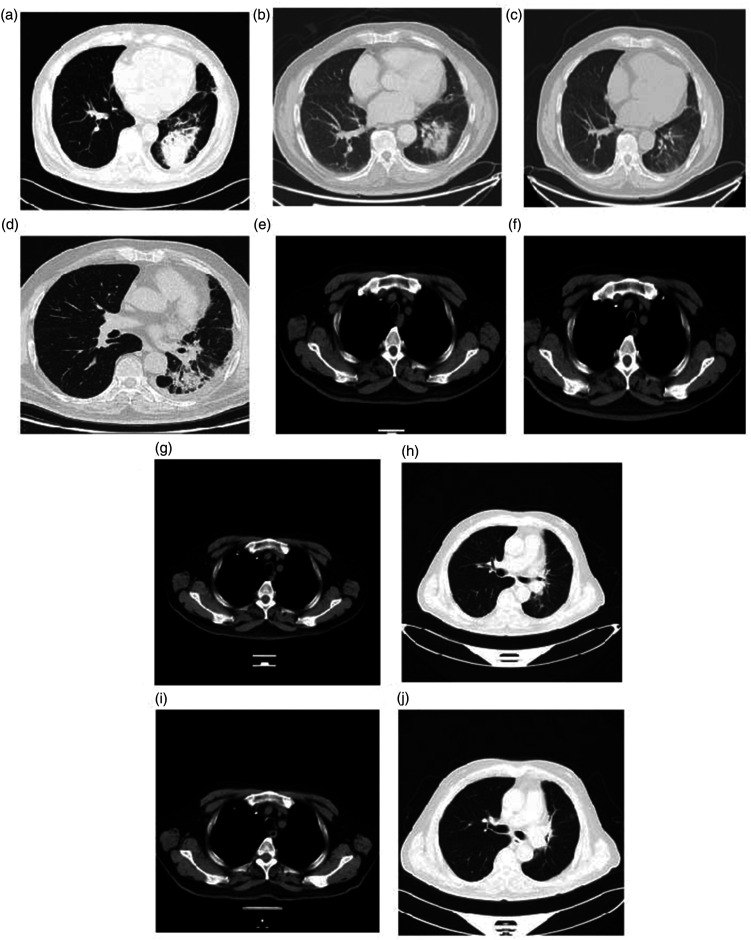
(a) Baseline chest CT scan in August 2019. (b) Chest CT scan after two cycles of etoposide combined with platinum treatment in September 2019. (c) Chest CT scan after four cycles of chemotherapy and CRT in November 2019. (d) Chest CT scan 6 months after CRT showing a new shadow in May 2020. (e) Chest CT showing a new lesion in a mediastinal lymph node in March 2021. (f) Chest CT after two cycles of paclitaxel (albumin-bound) and carboplatin combined with the PD-L1 inhibitor durvalumab in April 2021. Chest CT showing (g) a new lesion in mediastinum and (h) new lesions in the hilum and lower lobe of left lung in October 2021. (i) and (j) Chest CT after four cycles of EP combined with the PD-L1 inhibitor durvalumab in February 2022.
